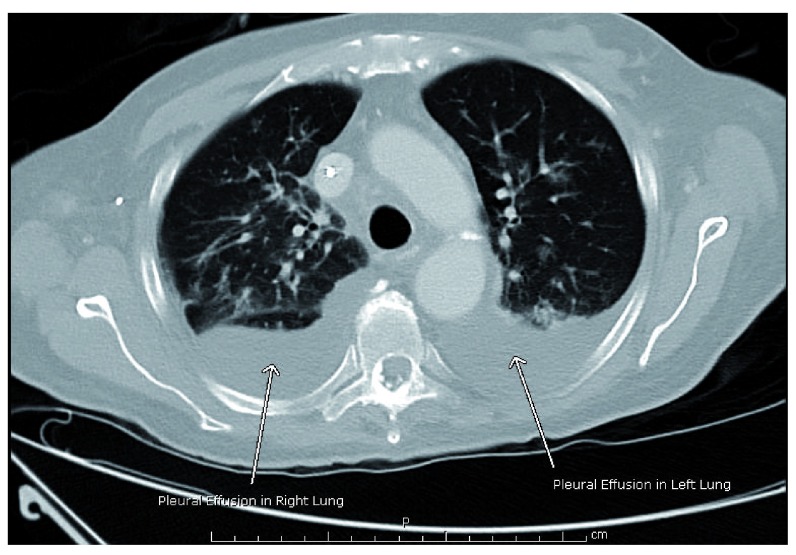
CT of the chest during hospitalization. CT of the chest showing nodular opacities that persisted in both lung fields and worsening bilateral pleural effusions (arrows).
